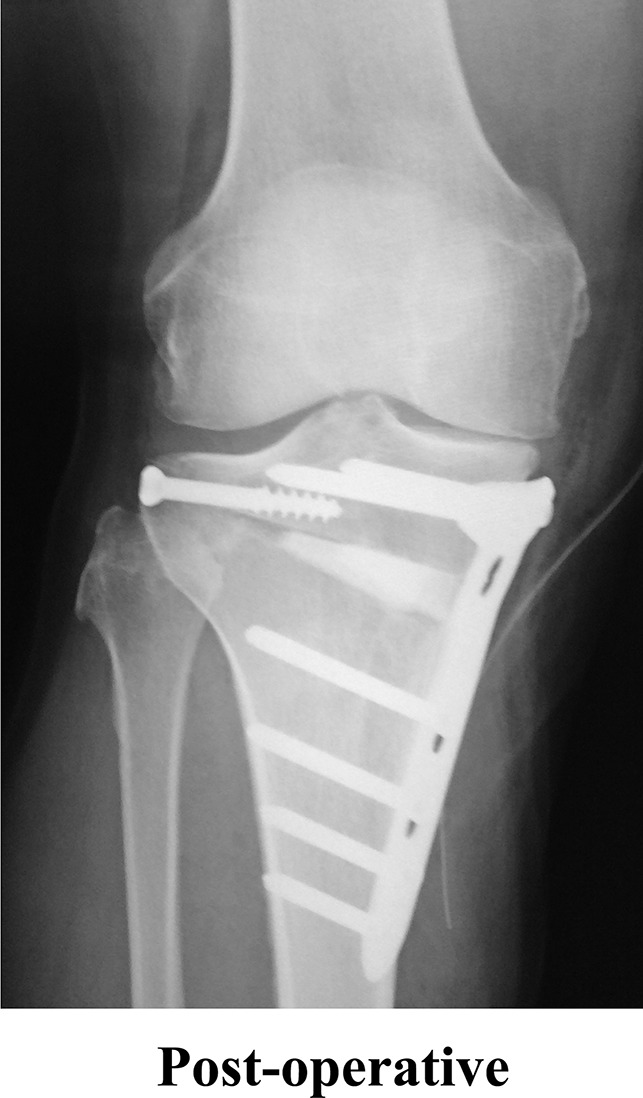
Right knee radiographs after surgery.
